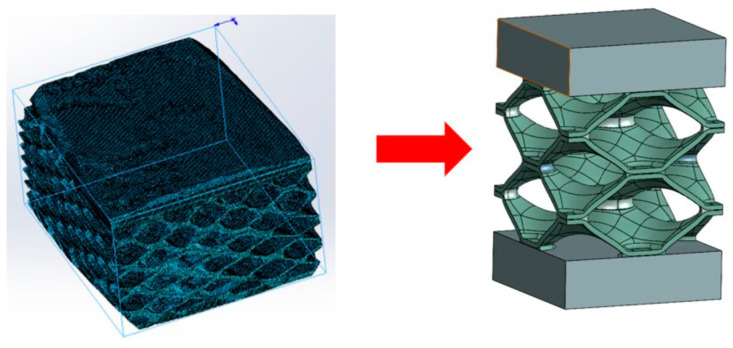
CT geometry (left) and simplified unit cell model (right).
